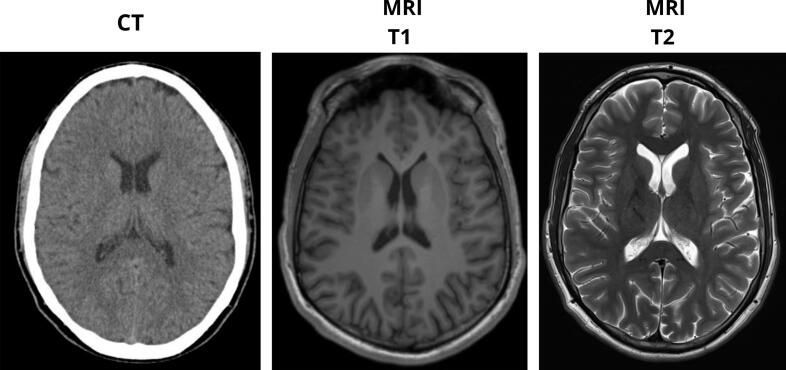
Neuroimaging during the diagnostic workup of exercise-induced seizures. Representative axial slices from brain imaging studies performed after the second seizure episode: non-contrast computed tomography (CT), and magnetic resonance imaging (MRI) T1- and T2-weighted sequences. All neuroimaging results were unremarkable, showing no structural abnormalities.
